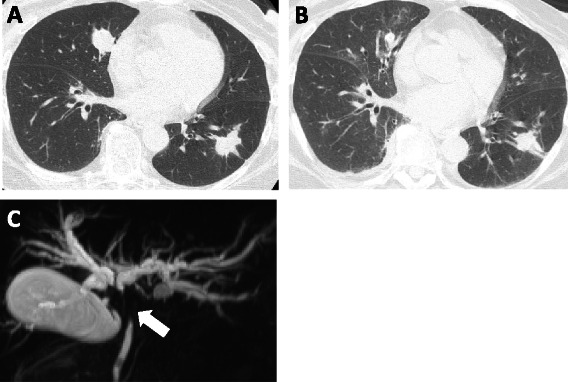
Images in clinical course: a Chest CT scan of lung metastases before introduction of sunitinib and gemcitabine. b Chest CT scan of lung metastases on day 20 after the administration. c Magnetic resonance cholangiopancreatography image demonstrated narrowing from upper common bile duct to bilateral intrahepatic bile ducts (arrow)
